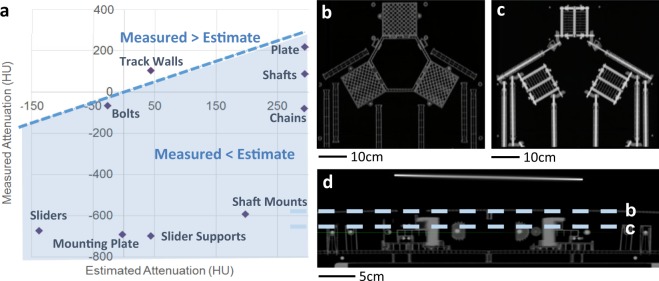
Validation of radio-compatibility. (a) Comparison between estimated attenuation and measured attenuation of materials used in the system. (b) Horizontal CT slice through the base of the system. (c) Horizontal slice through the vertical height of the axles and gears. (d) Side-view of radiographic image, dashed lines indicate vertical heights of slices shown in (b,c). These sectional CT images show no visible artifacts.
